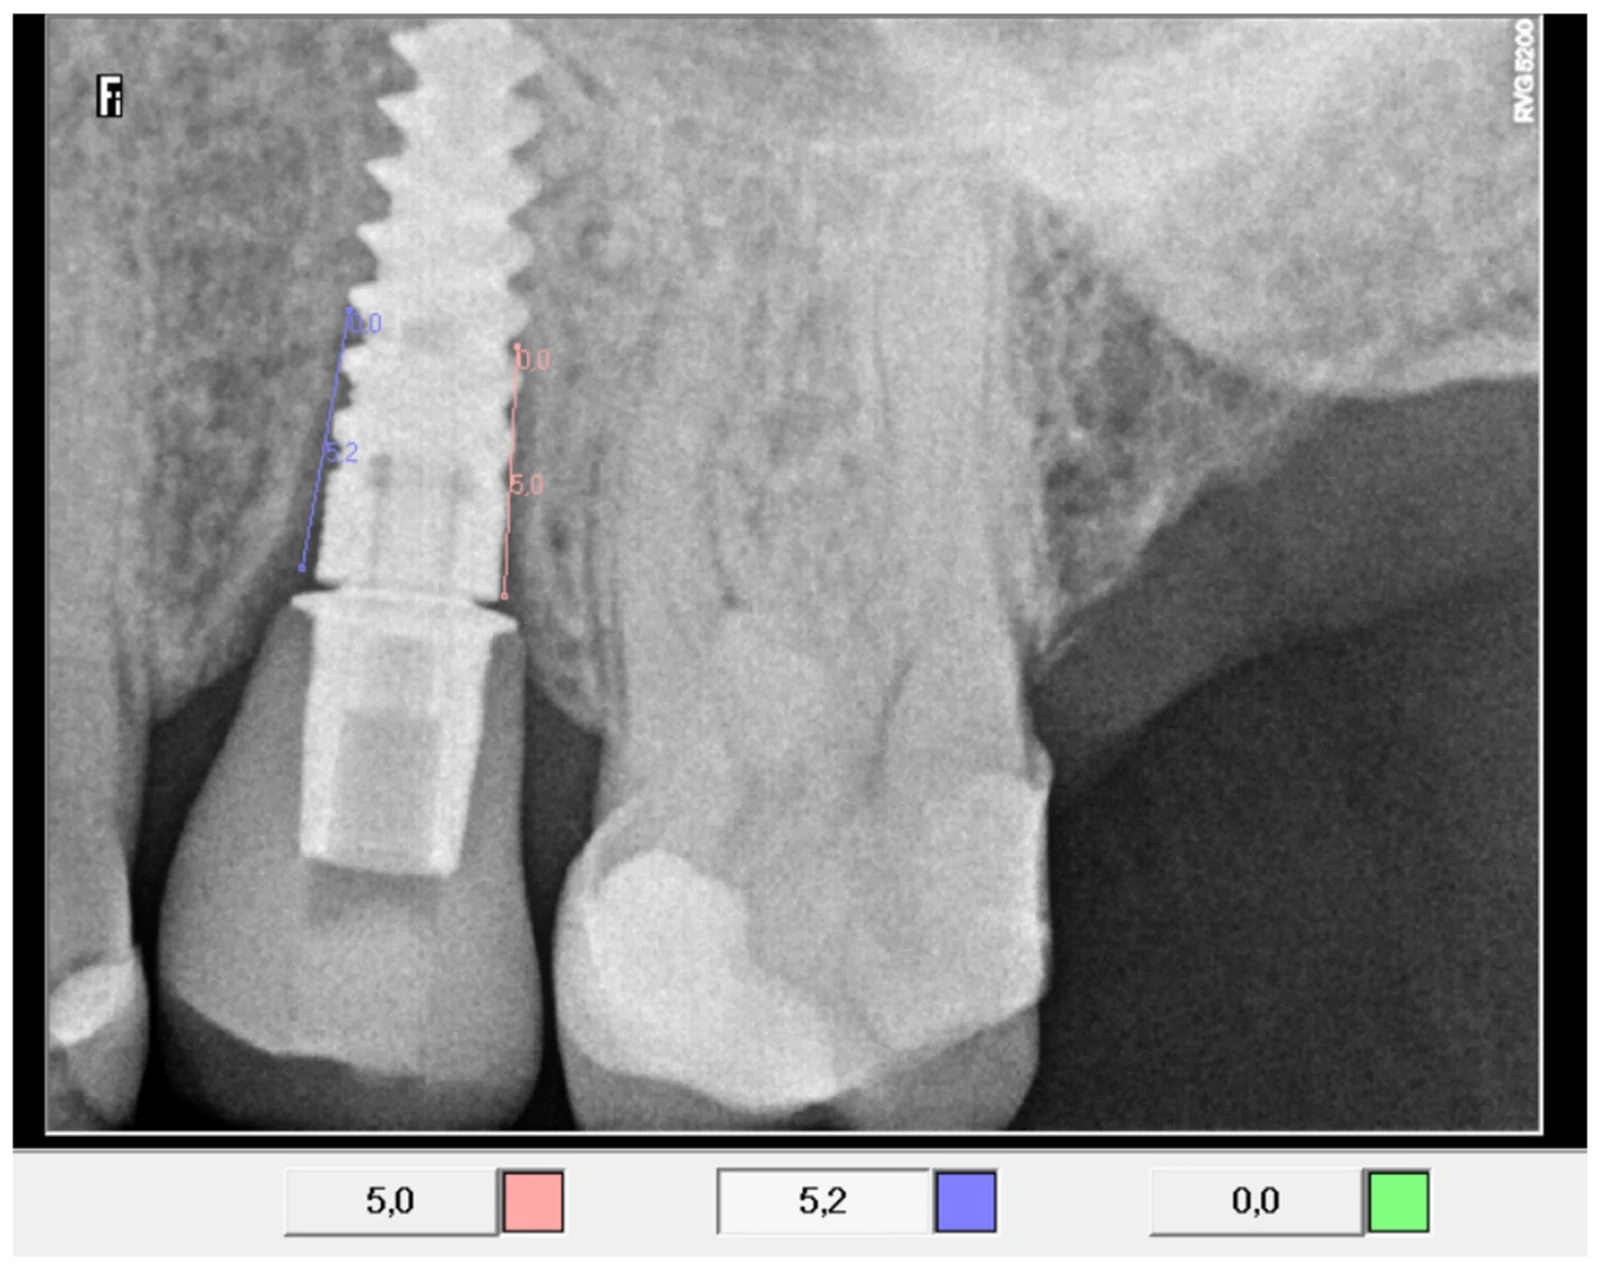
Radiograph of the implant in position 25 obtained at the initial follow-up visit.
